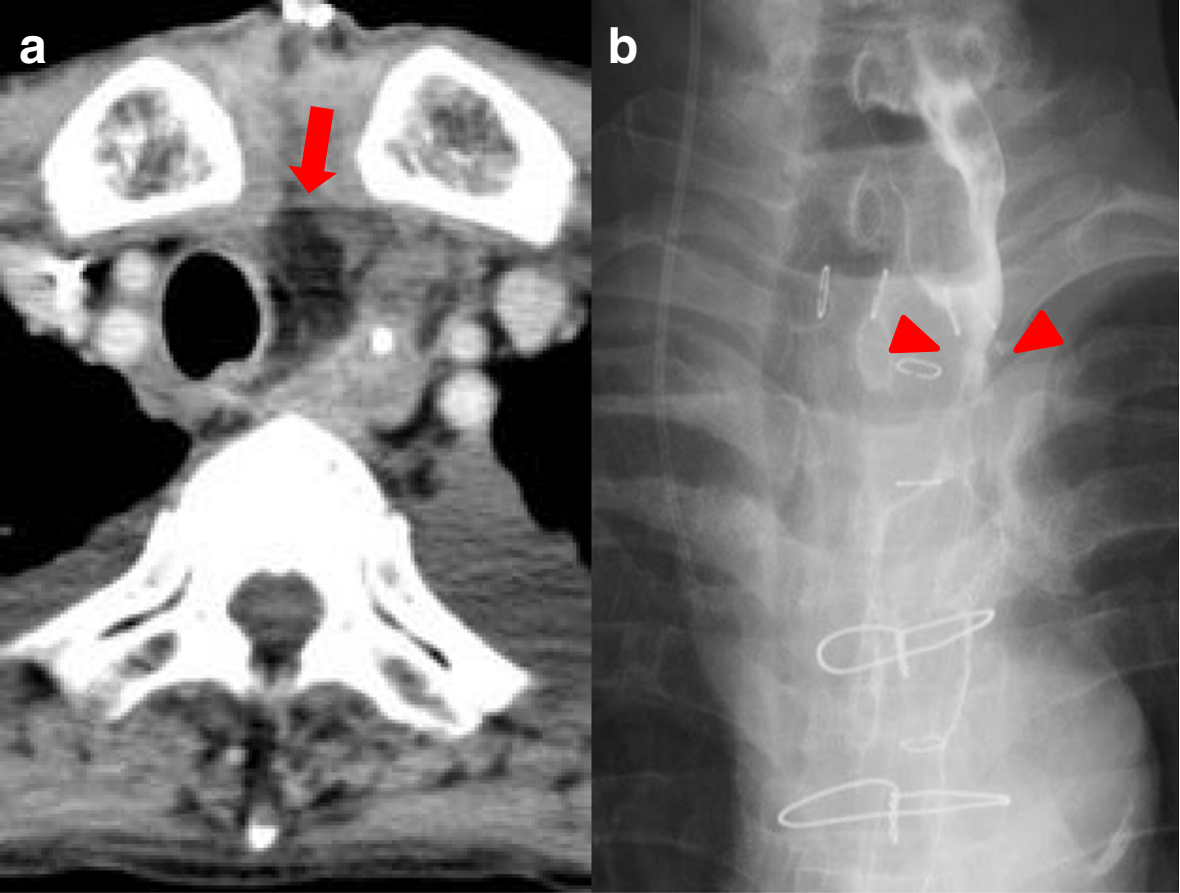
The findings after surgery for tracheoesophageal fistula (TEF). a CT image. The arrow indicates the thymus pedicle flap. b Esophagography shows no leakage on the esophagogastric anastomotic site (arrowheads)
